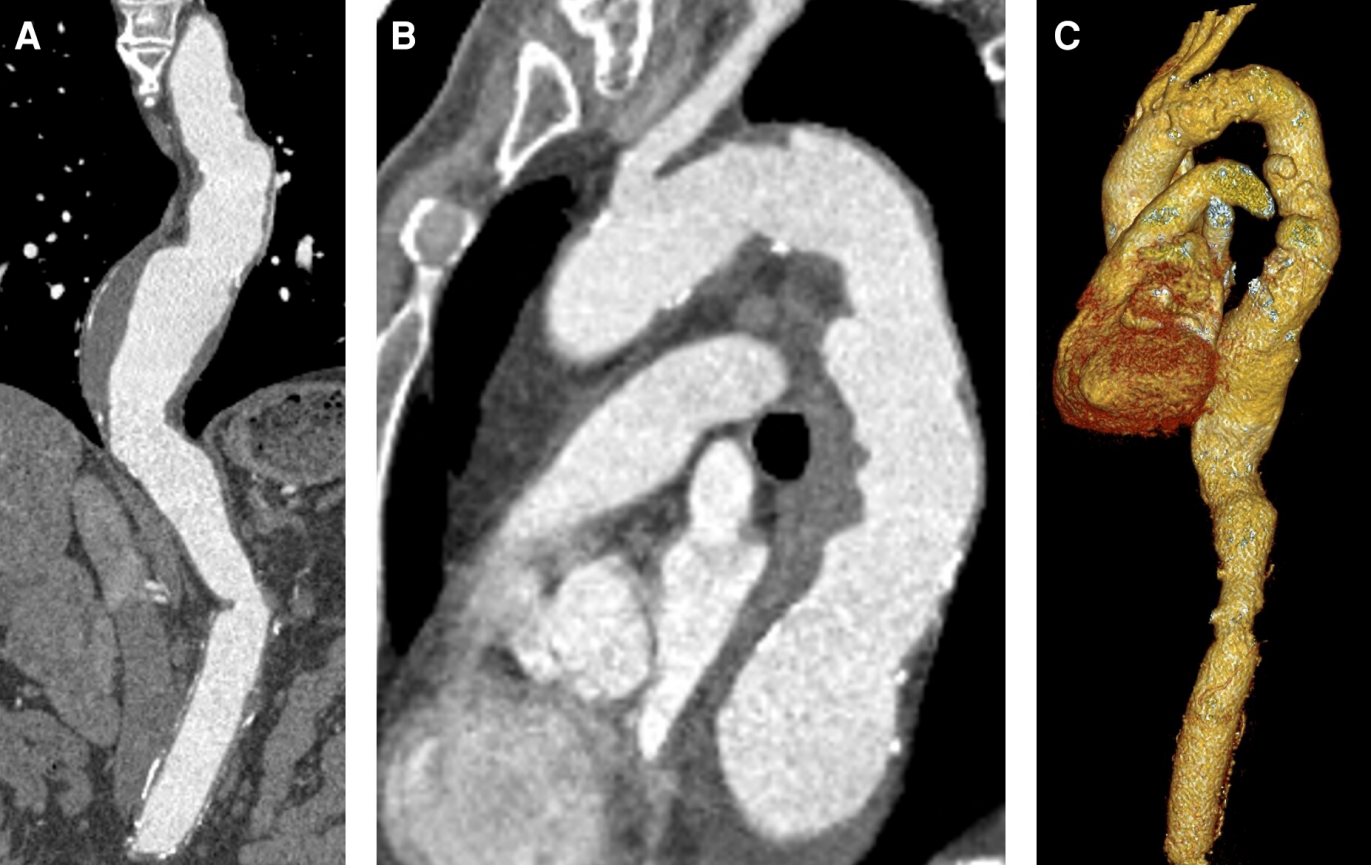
Preoperative CTA. (A) Descending aortic aneurysm with 63 mm diameter. (B) Proximal landing zone distal to the left subclavian artery (30 mm). (C) 3D reconstruction.
